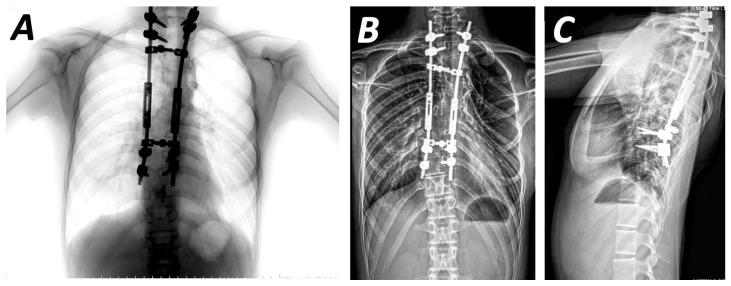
Postoperative radiographs of juvenile patient with NF1 after the lengthening of the growing rods that resulted in the correction of the deformity at the range of 16° (A). The 10 years of follow -up demonstrated maintenance of the correction without any clinical or imaging progression of the curve (B,C).
